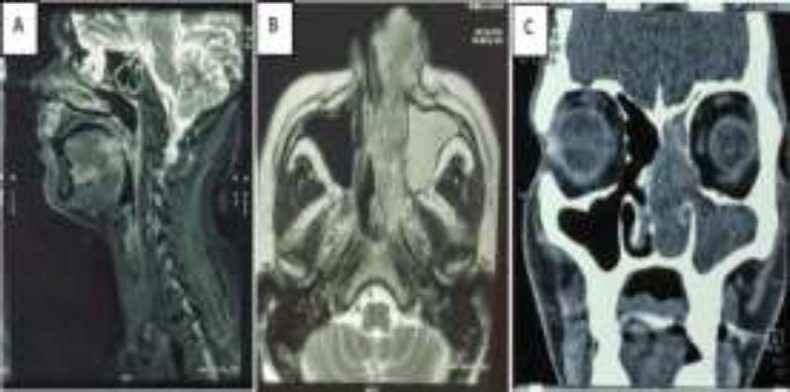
Radiological investigations. Image 3A showing Sagittal section MRI with a visible lesion involving the tongue base. Image 3B is an axial cut of MRI showing an irregular heterogeneously lobulated elongated soft tissue mass in the left nasal cavity extending onto deeper fascial planes at the medial canthus of the left orbit and into the left nasopharynx. Also visible is the mucosal thickening occupying the whole of the left maxillary sinus. Image 3C shows coronal section image of CECT Nose-Paranasal Sinus with evidence of a soft tissue mass filling the entire left nasal cavity. There is a widening of left osteo-meatal complex, bowing of septum to the right side due to mass effect of the lesion. The left side lamina papyracea appears to be eroded
